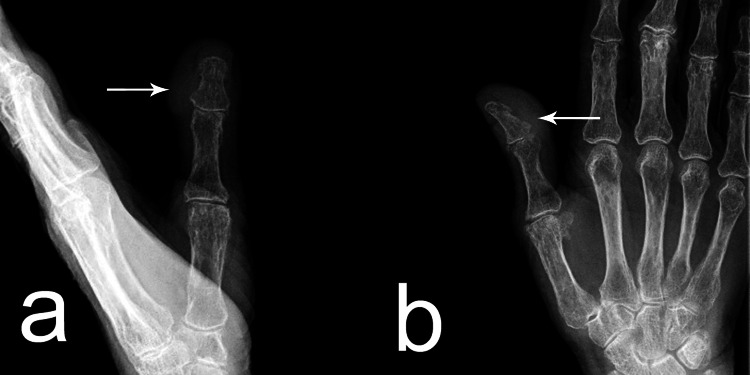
Roentgenography presented a clearly defined soft tissue formation in the thumb area without involvement of bone structures (a – anterior-posterior view; b - lateral view).
